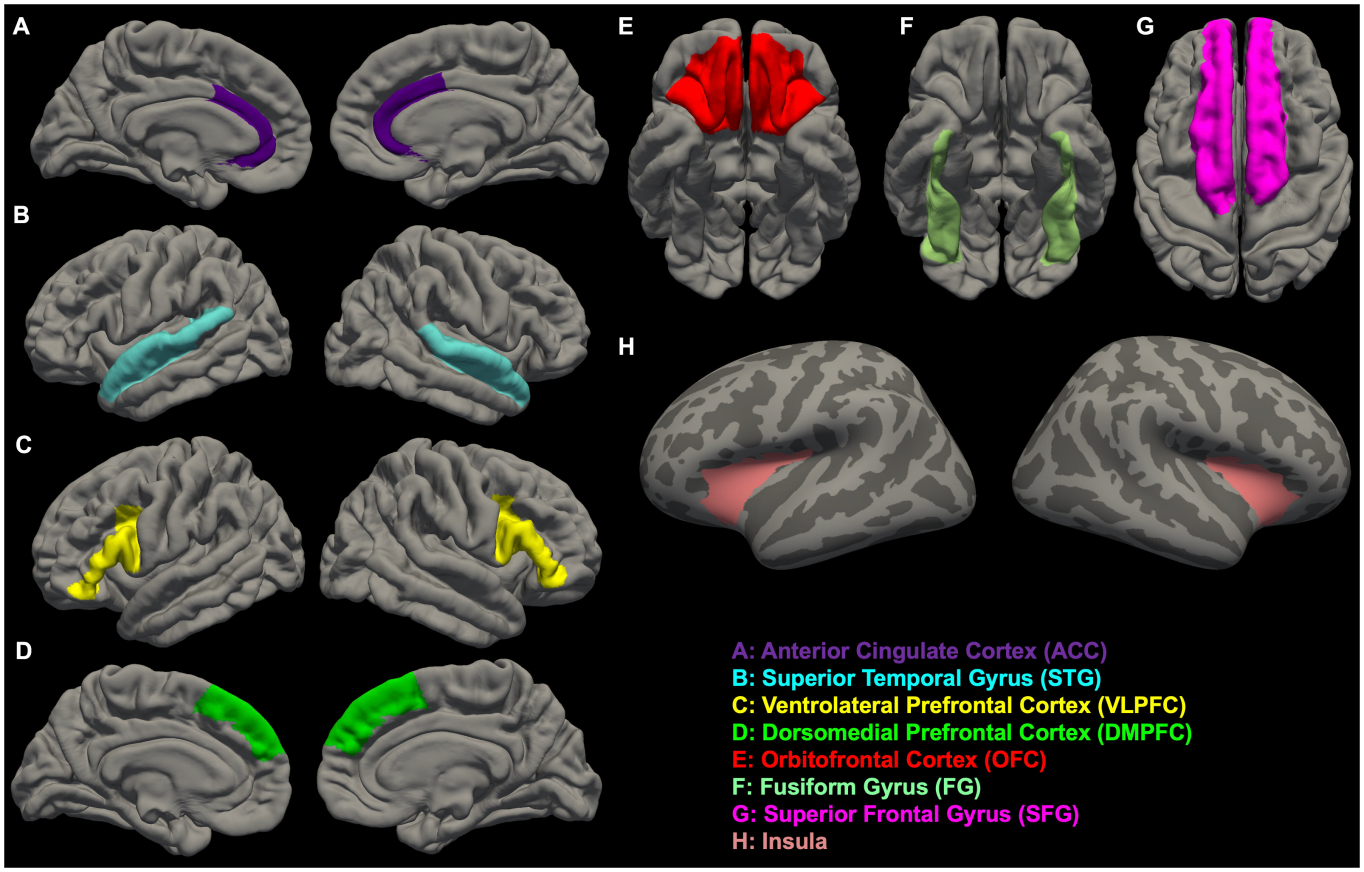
Overview of brain regions (A–H) that are most commonly found to be involved in functional MRI and brain morphometry research of suicidal thoughts and behavior.
